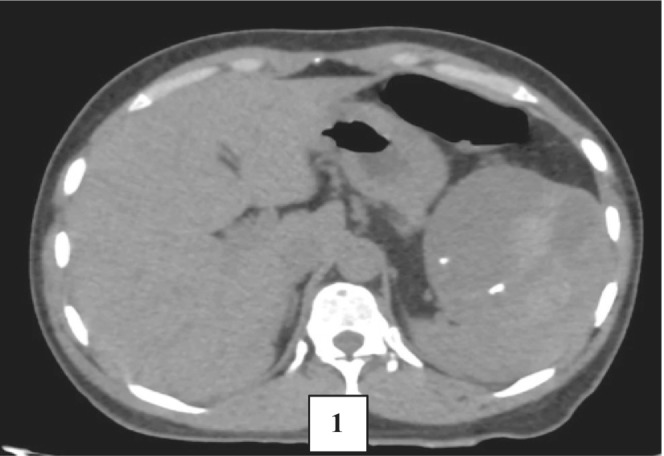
Plain axial CT of the cyst showing areas of relative hyperdensity and speckled calcification within.
